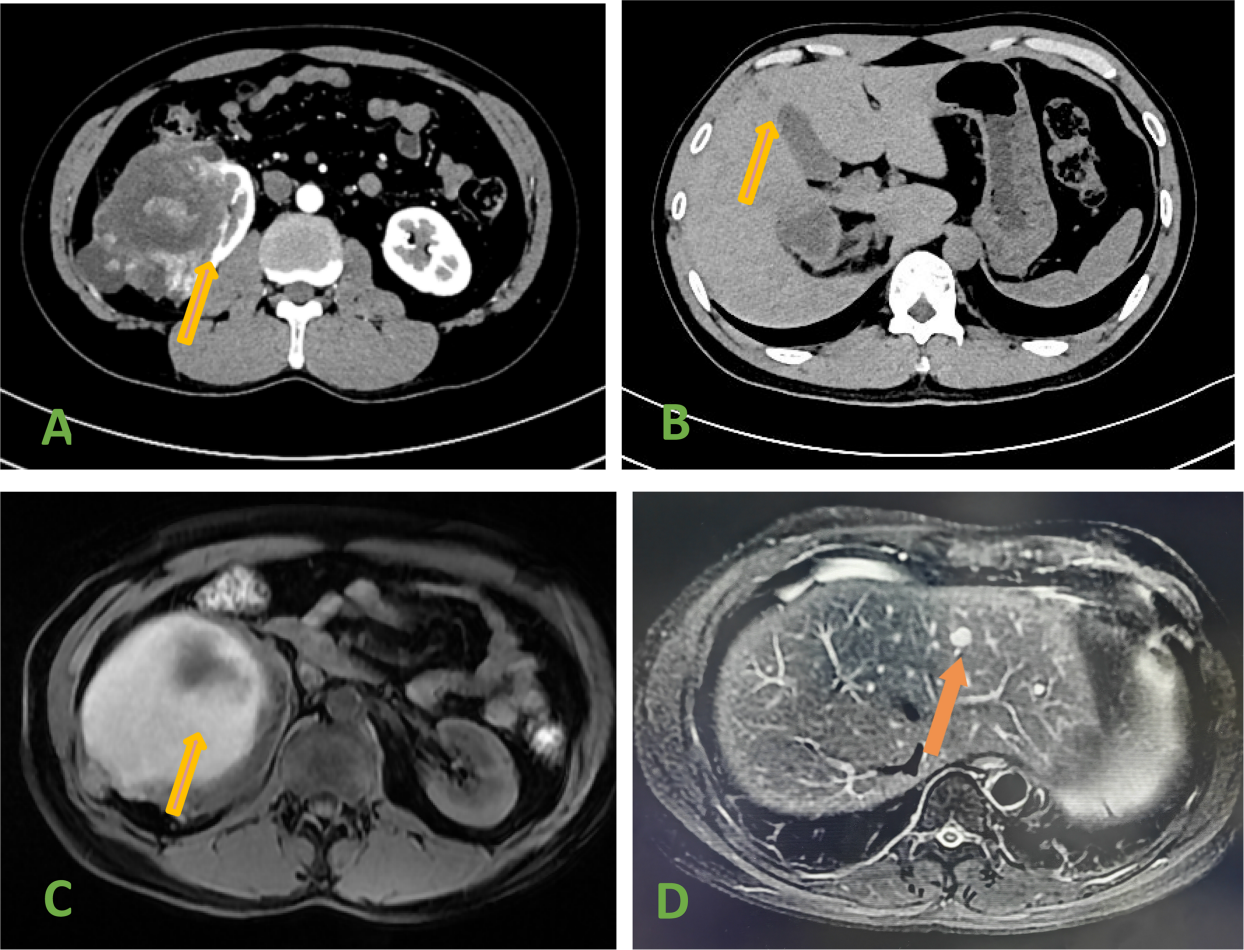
(A) Ct scan showed a tumor in the right kidney. (B) CT scan showed hepatic metastases. (C) MRI showed a tumor in the right kidney. (D) MRI Showed hepatic metastases (arrow).
